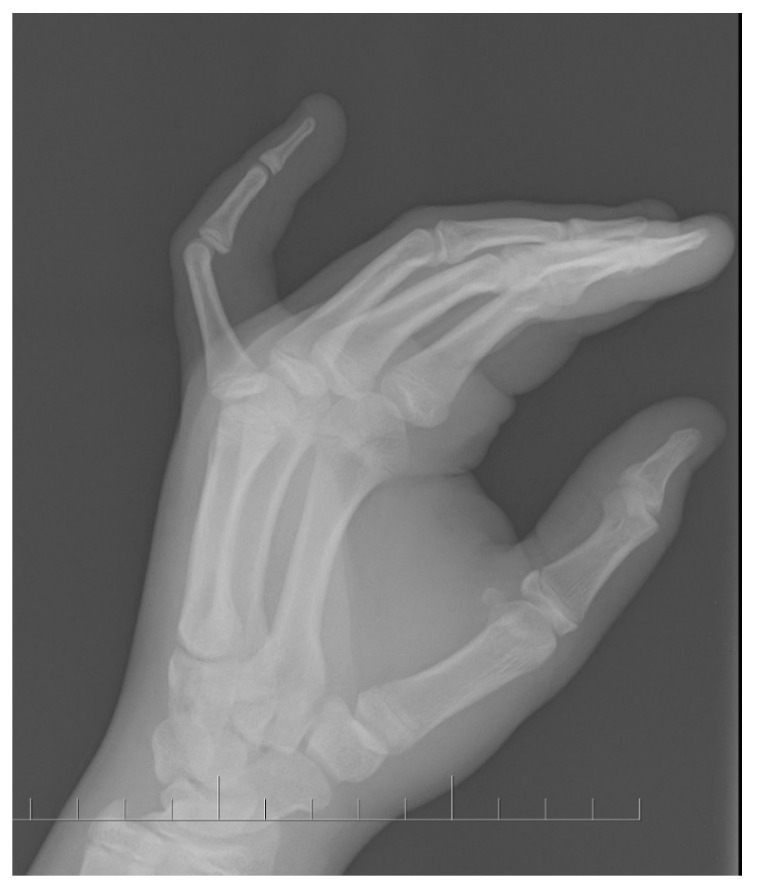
Lateral X-ray of the left hand with 60-degree PIPJ contracture.
